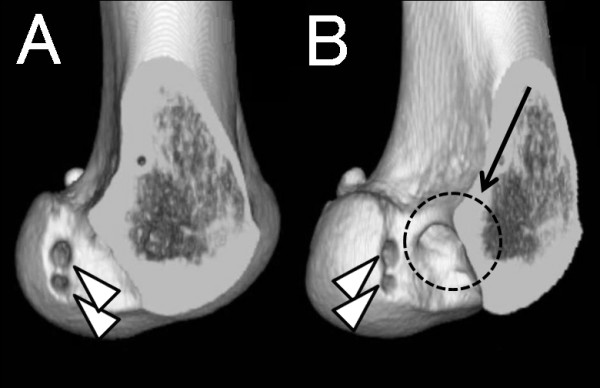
Postoperative 3-D CT image after the anatomic double-bundle revision ACL reconstruction. From the medial view (A) and posteromedial view (B). Original high noon tunnel (arrow) and two new anatomically placed femoral tunnels for AM and PL bundles (arrowheads) are shown.
